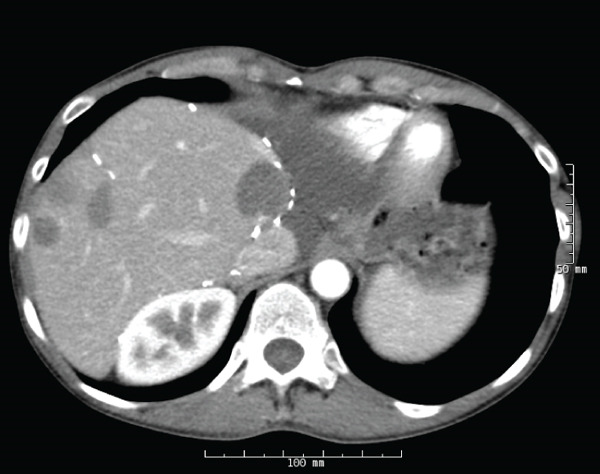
Surveillance CT abdomen and pelvis showing progression of hepatic metastases including a 1.5 × 1.4 cm subcapsular Segment VI lesion.
